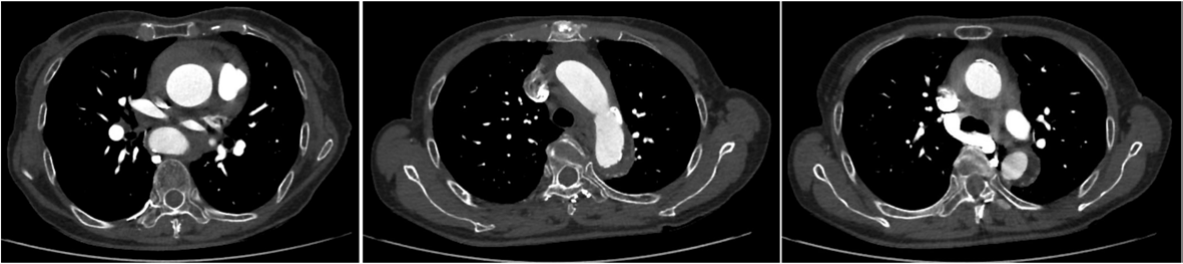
CT findings after emergent transportation. CT shows no primary entry and a thrombosed false lumen from the ascending to descending aorta. CT, computed tomography
